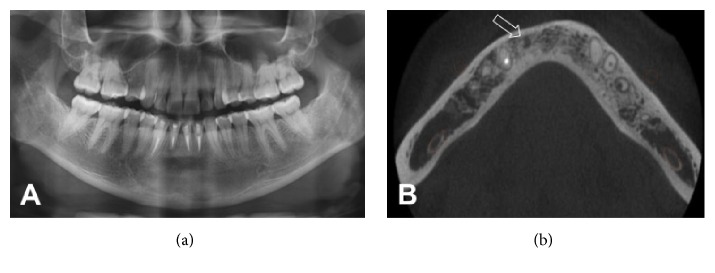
(a) Panoramic radiograph obtained at 4-year follow-up. Bone repair is clearly visible; (b) cone-beam computed tomography image (axial slice), demonstrating bone repair. A possible fibrotic bone scar is visible (arrow).
